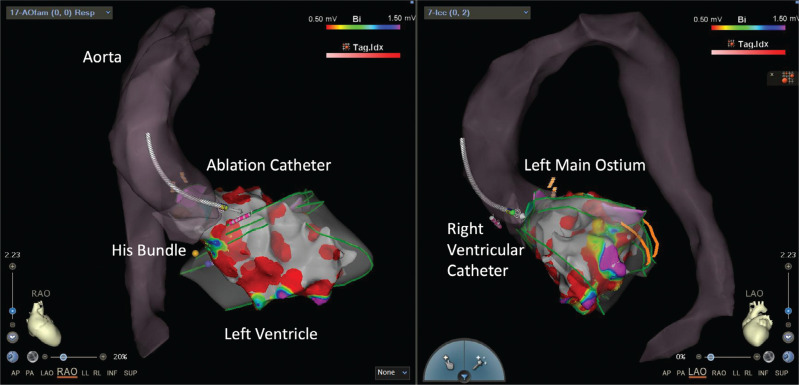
Electroanatomic map in a patient undergoing left ventricular tachycardia ablation. The map was guided by an ultrasound shell created earlier using intracardiac echocardiography.
